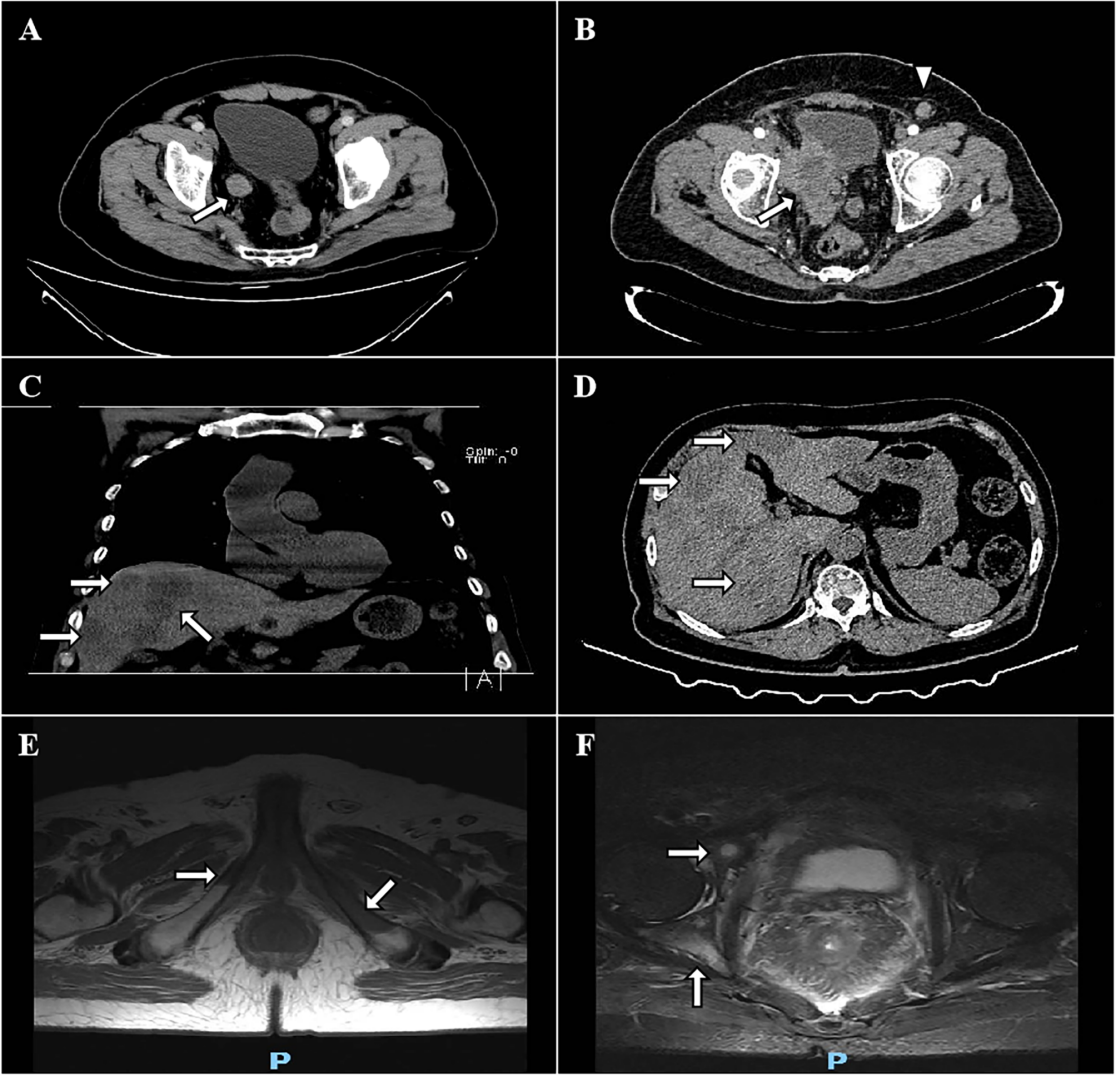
CT and MRI images of the mass. The preoperative transverse view reveals that the tumor was located in the lower segment of the right ureter (A). CT scan reveals local relapse in the tumor bed with infiltration to the surrounding tissues (white arrow, B). Left inguinal lymphadenopathy (2.1x1.5 cm, white triangle, B). Coronal and transverse views show a progressive SCNEC with multiple liver (C, D), bilateral pubic bones (E), and right acetabulum (F) metastases after 2 cycles of durvalumab.
